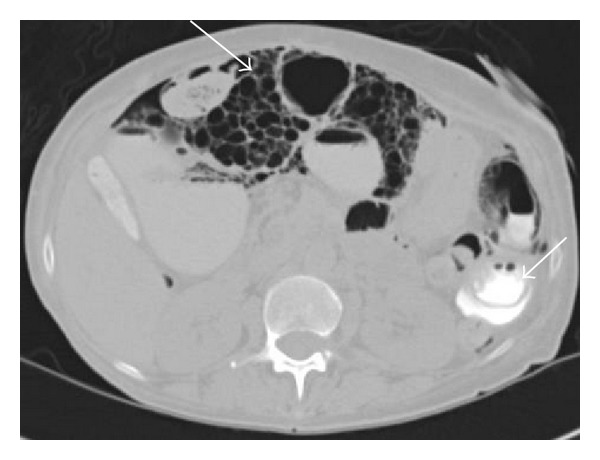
Axial CT image with enteric contrast demonstrates multiple large “cysts” within the wall of the small bowel (arrows). Enteric contrast is visualized within the lumen of small bowel at the left side of the abdomen, without extravasation (dashed arrows).
